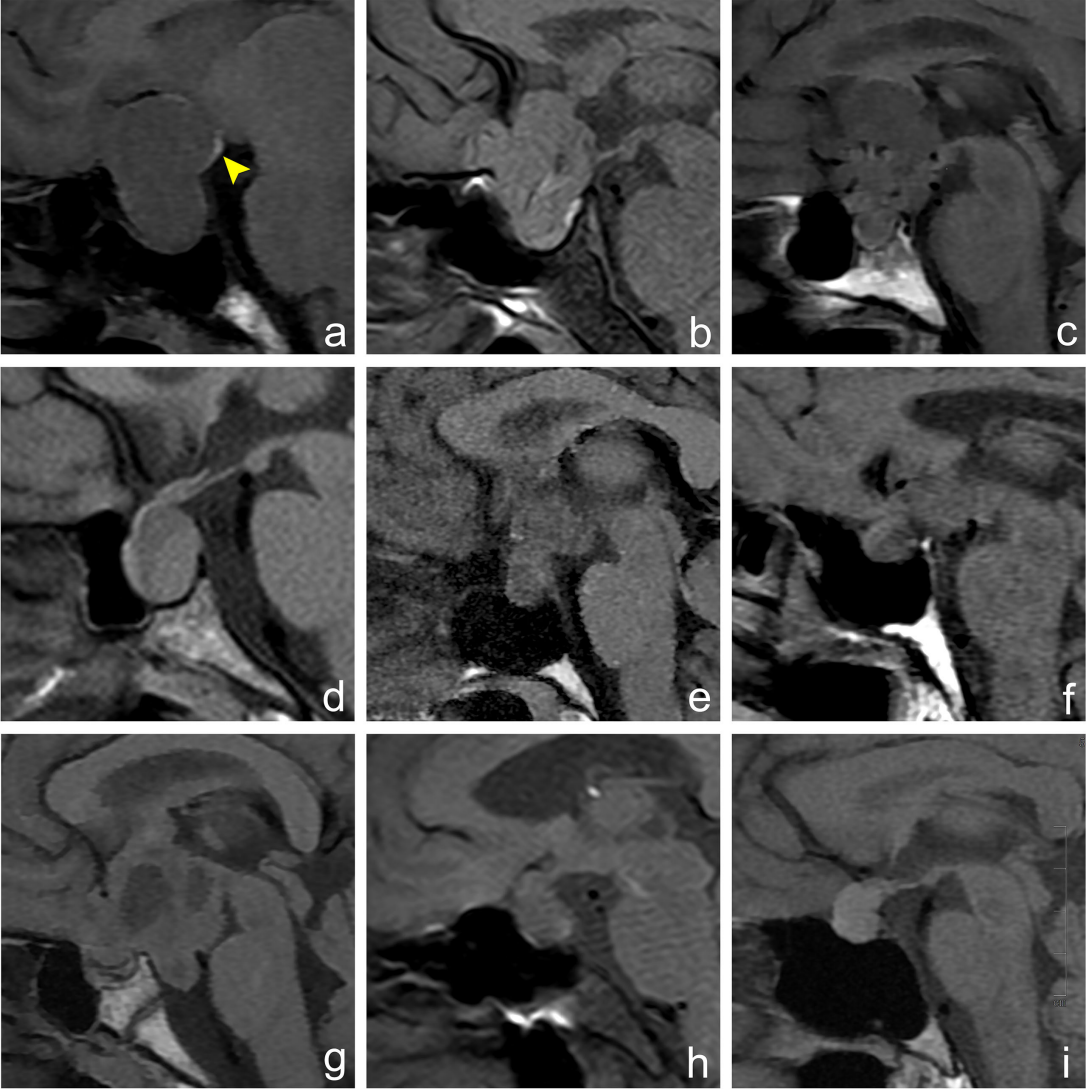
Imaging manifestations of hyperintense signal in posterior pituitary in different sellar diseases. Figures (A–I) show an MRI sagittal T_1_WI non-enhanced scan of different lesions in the sellar region. (A) A 30-year-old female, pituitary macroadenoma, ectopic hyperintense signal in the posterior pituitary, which is located at the posterior upper edge of the tumor (arrowhead). (B) A 64-year-old male, meningioma, normal hyperintense signal in the posterior pituitary, which is located behind the intrasellar adenohypophysis. (C) A 39-year-old male, craniopharyngioma, hyperintense signal in the posterior pituitary disappears. (D) A 54-year-old female, Rathke cleft cyst, hyperintense signal in the posterior pituitary disappears. (E) A 35-year-old female, germ cell tumor, hyperintense signal in the posterior pituitary disappears. (F) A 60-year-old male, metastatic tumor, hyperintense signal in the posterior pituitary disappears. (G) A 14-year-old female, glioma, hyperintense signal in the posterior pituitary disappears. (H) A 56-year-old female, cryptococcal granuloma of pituitary, hyperintense signal in the posterior pituitary disappears. (I) A 40-year-old female, abscess of the pituitary, hyperintense signal in the posterior pituitary disappears.
